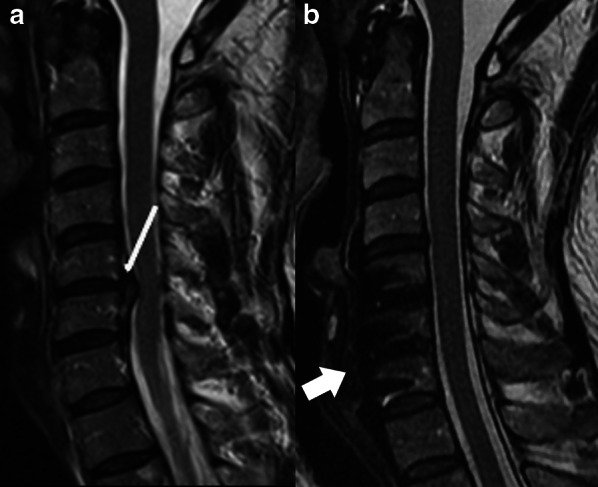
Plate with screws—anterior fusion procedure for degenerative disc disease. A disc herniation at the C5-6 level (thin arrow) compressing the spinal cord is demonstrated on a preoperative T2W sagittal MR image (a). An anterior cervical fusion procedure was performed from C5 to C7 using plate with screws attached to the vertebral bodies (arrow), as shown on a postsurgical T2W sagittal MR image (b). Resection of the herniated disc material was performed
